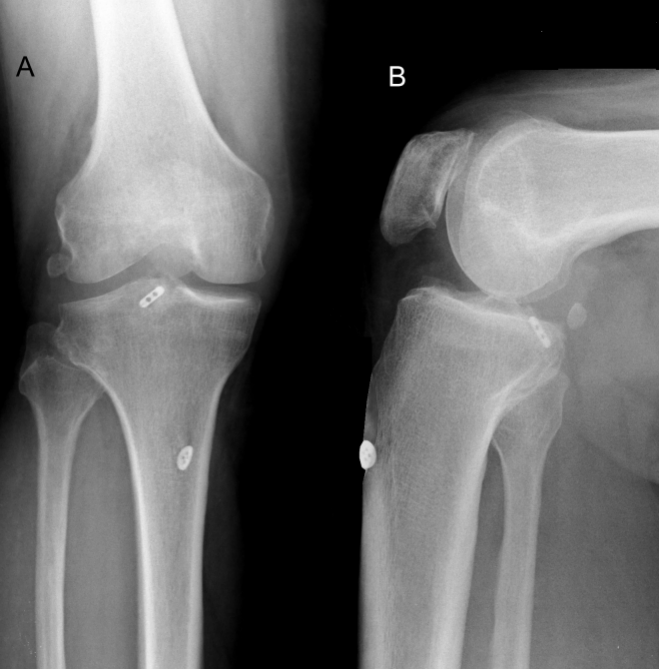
Anteroposterior (A) and lateral (B) radiographs six month after surgery showing anatomically reduction and union of the bony avulsion
